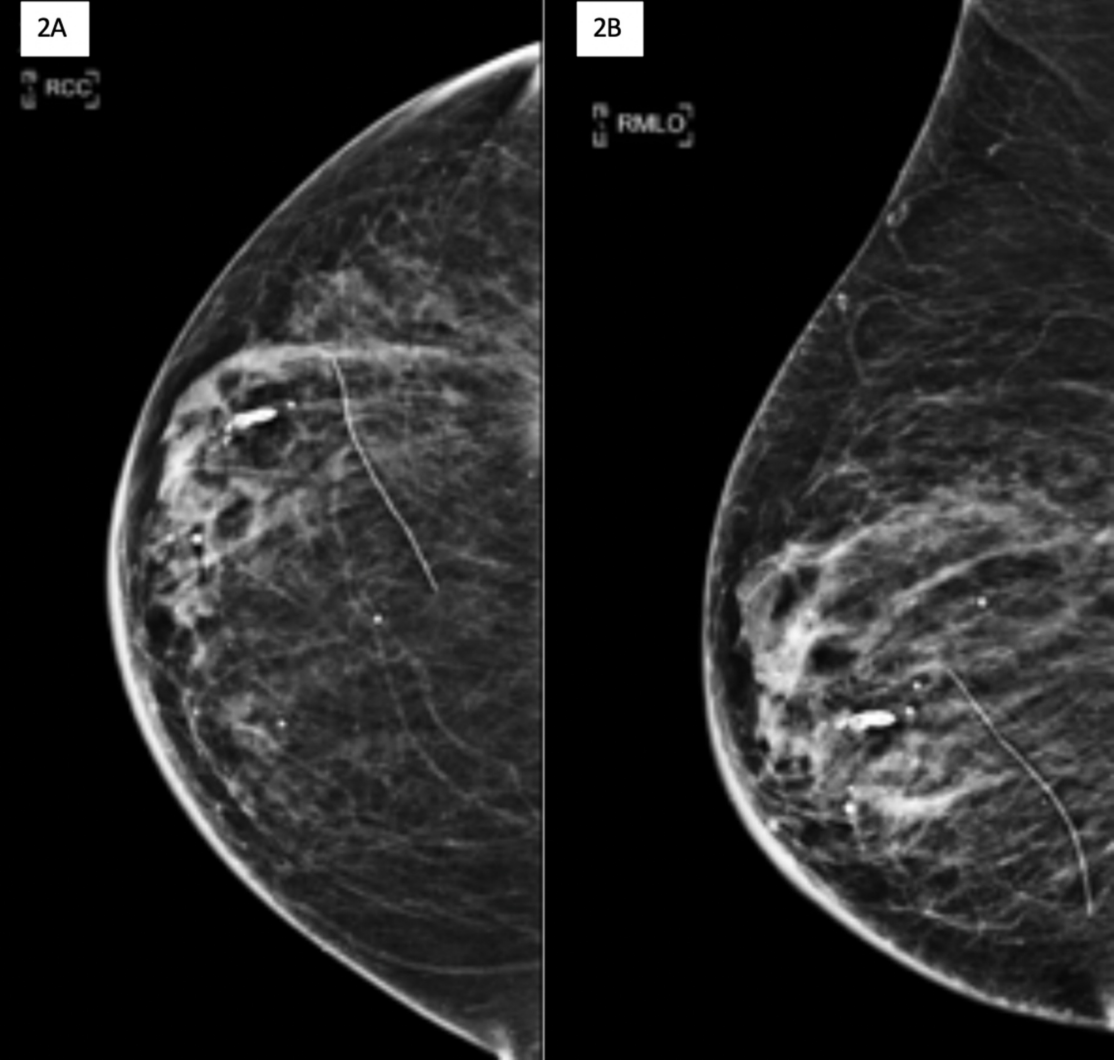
Most recent screening mammogram two weeks prior to the abnormal CT scan Right craniocaudal (A) and mediolateral oblique (B) views from most recent mammogram. There are postlumpectomy changes with no suspicious masses, calcifications, or abnormal findings. The abnormal CT finding was far posterior and thus not included in the field of view on mammogram.
